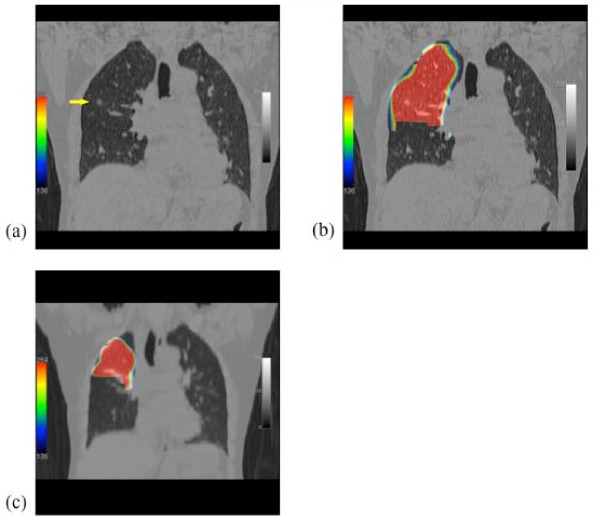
Images of before and after segmentectomy. (a) Coronal image of CT before surgery, showing a lung cancer (arrow) in the segment 2b of right upper lobe. (b) Coronal image of the perfusion SPECT/CT of the right upper lobe before operation. (c) Coronal image of the perfusion SPECT/CT of the remaining right upper lobe after the resection of S2b and S3a.
